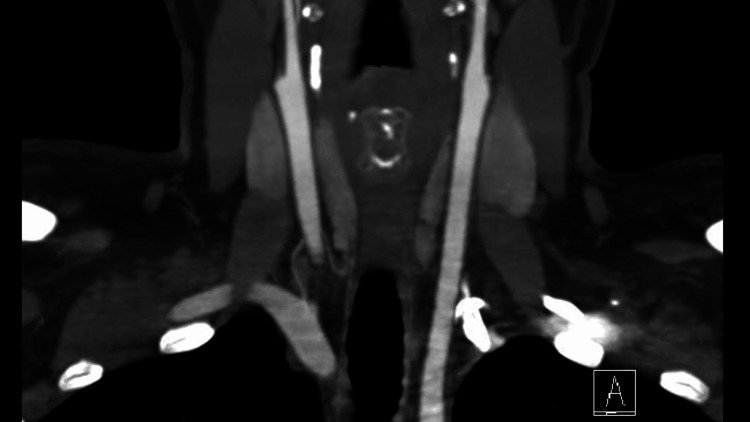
Follow-up CT angiography.
